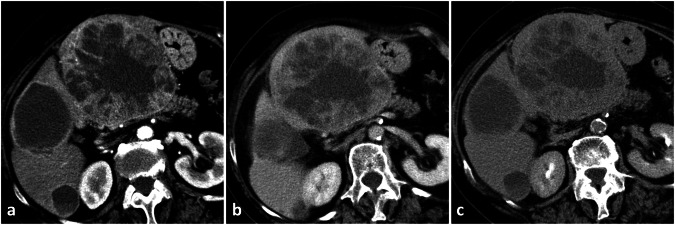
Hemangioendothelioma (biopsy-proven). Three markedly hypodense lesions with moderate peripheral enhancement are appreciable in late arterial phase CT (a); mild progressive centripetal filling is recognizable in portal venous (b) and delayed (c) phase, with persisting large hypodense areas
